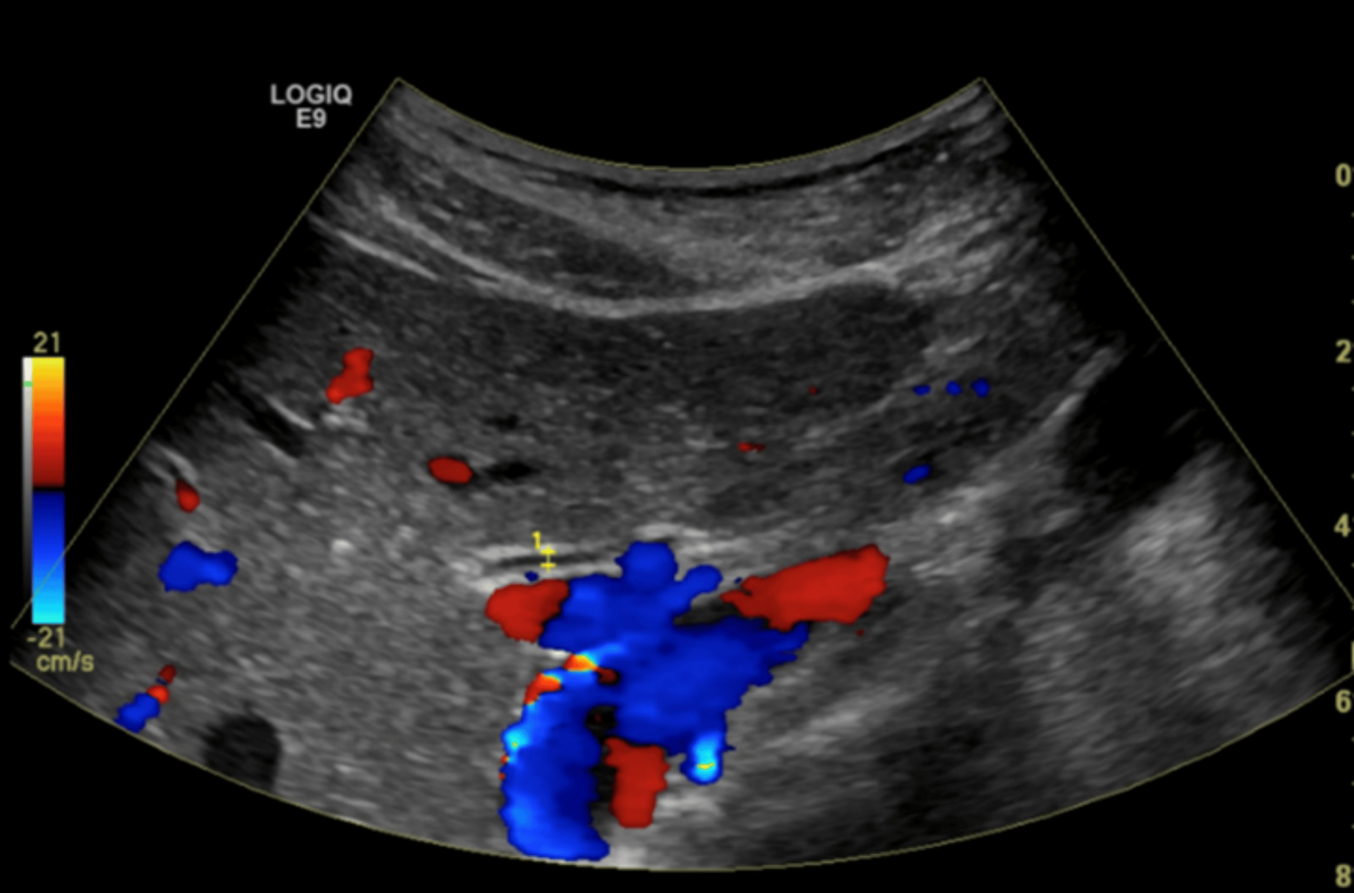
Ultrasound of the right upper quadrant (RUQ) reveals no dilation to the common bile duct, which measures 5 mm.
